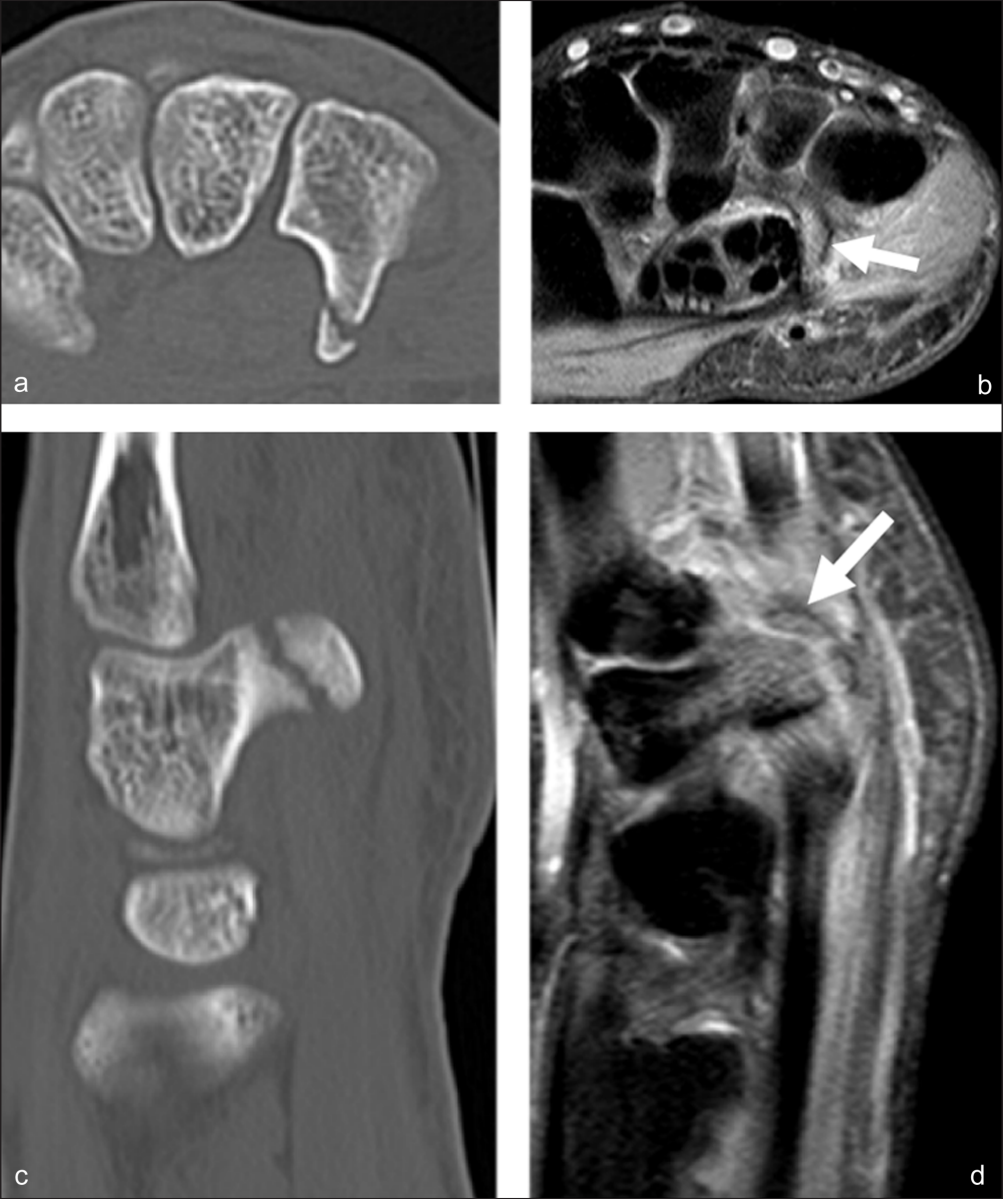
Another case of direct compression of the hook of the hamate. Axial CT (a) and sagittal reconstruction (c) disclose the avulsion fracture, much easier than the corresponding axial (b, arrow) and sagittal (d, arrow) proton-density fat-saturated MR images.
